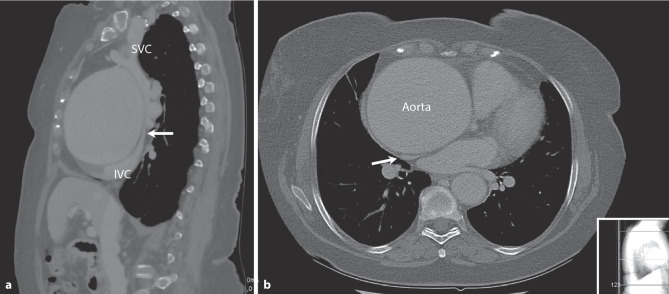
Computed tomography angiography of aorta, in a sagittal view and b transversal view. *Arrows* mark compression of both superior vena cava (*SVC*) and inferior vena cava (*IVC*)
